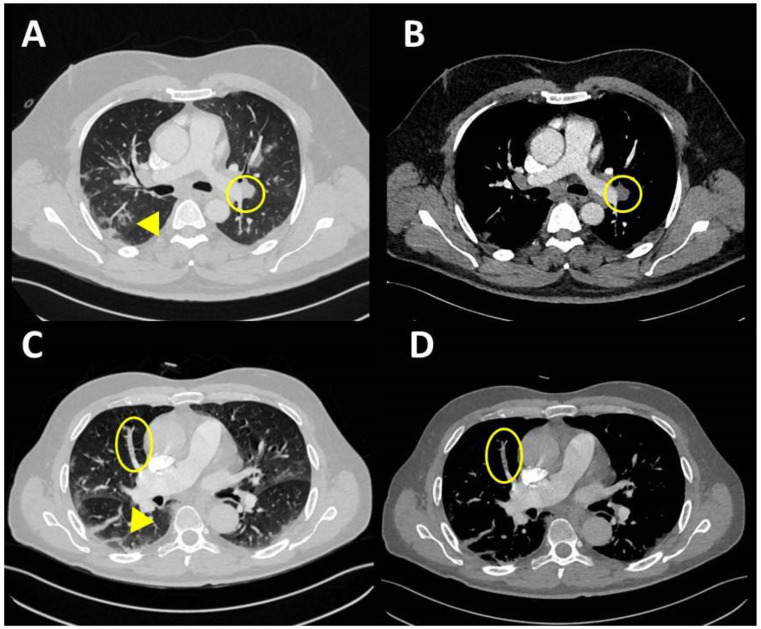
CT scan is able to identify both gross pulmonary embolism (A,B) and tinier obstructions in subsegmental artery (C,D). Embolism (Circles) is not related to site of pneumonia but to endotheliitis and not to pulmonary inflammation (Triangles).
